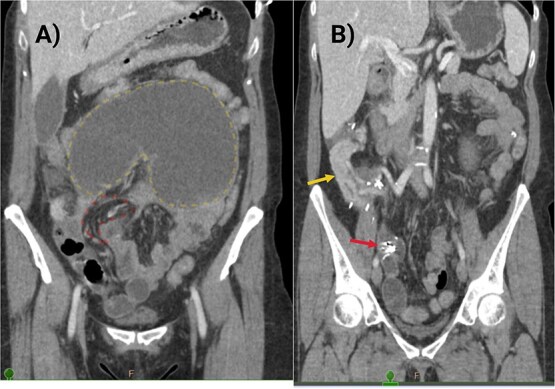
Coronal sections of intravenous contrast-enhanced CT showing (A) air-distended cecal loop/coffee bean sign, outlined by the large dashed contour, and mesenteric torsion/whirlpool sign, outlined by the smaller dashed contour, characteristic of cecal volvulus; and (B) new pancreas transplant (upper arrow) and previous pancreas transplant (lower arrow) in the right abdomen.
